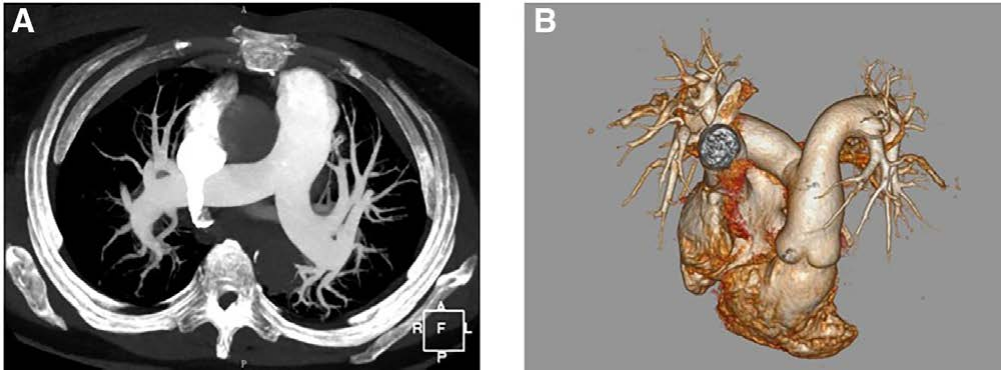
Pulmonary computed tomography angiography of the patient with pulmonary tumor thrombotic microangiopathy as the disease aggravated. (A) The diameter of the main pulmonary artery was widened (35 mm), suggesting pulmonary hypertension. (B) Three-dimensional reconstruction of pulmonary vessels indicated that there was no filling defect in the pulmonary artery.
